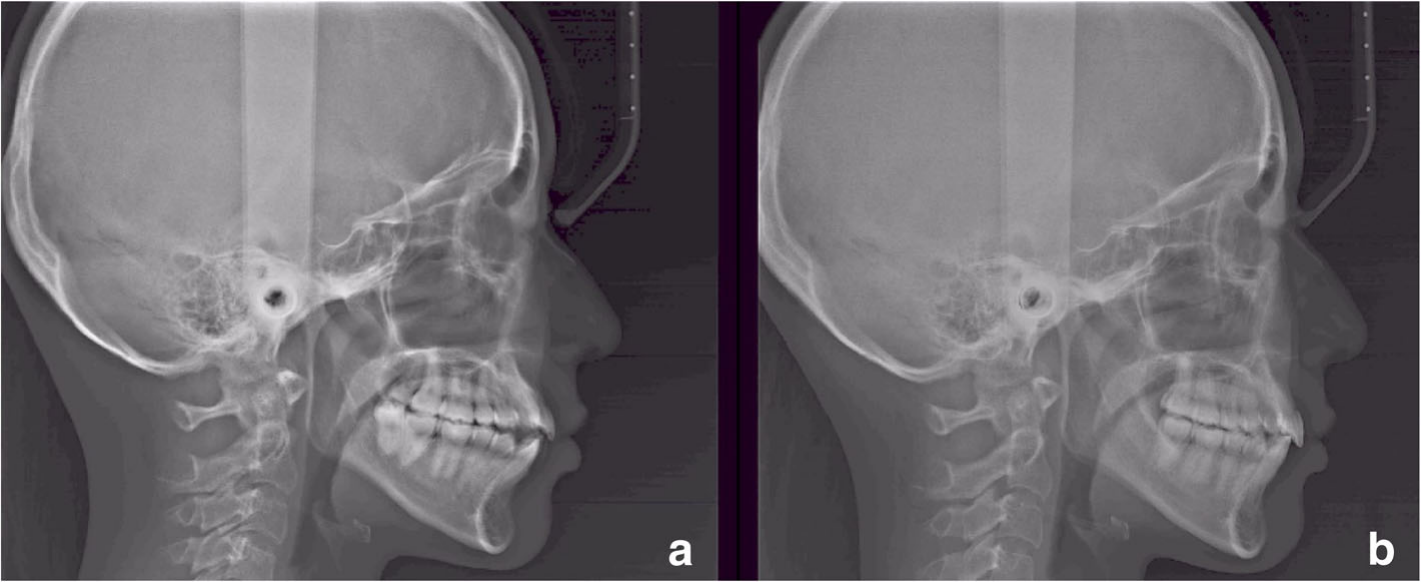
a-b: Lateral cephalometric radiographs of a patient, before the orthodontic treatment with sequential distalization (a) and after treatment (b)
